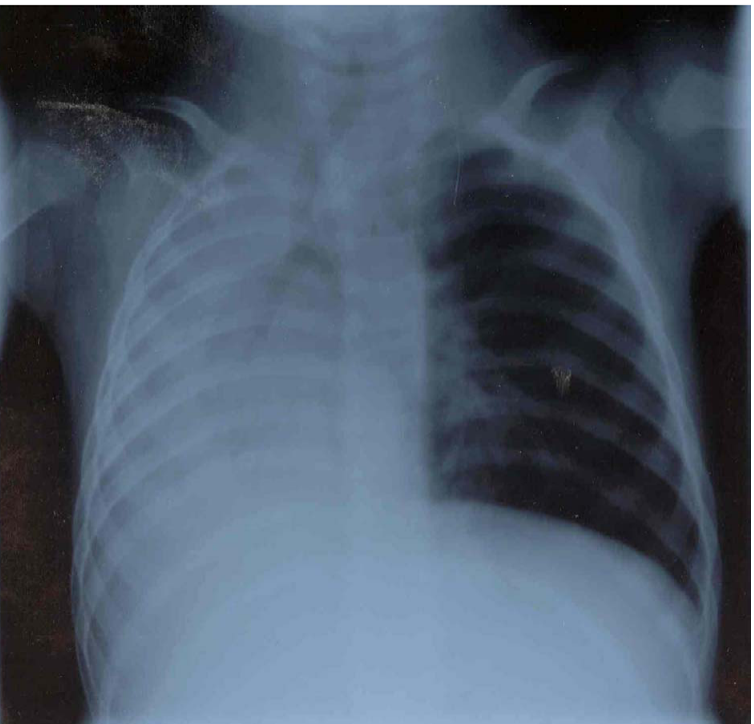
Chest X-ray shows persisting total atelectasis of the right lung. Note the marked loss of volume on the right, pronounced shift of the mediastinum to the right, and compensatory overexpansion of the left lung.
